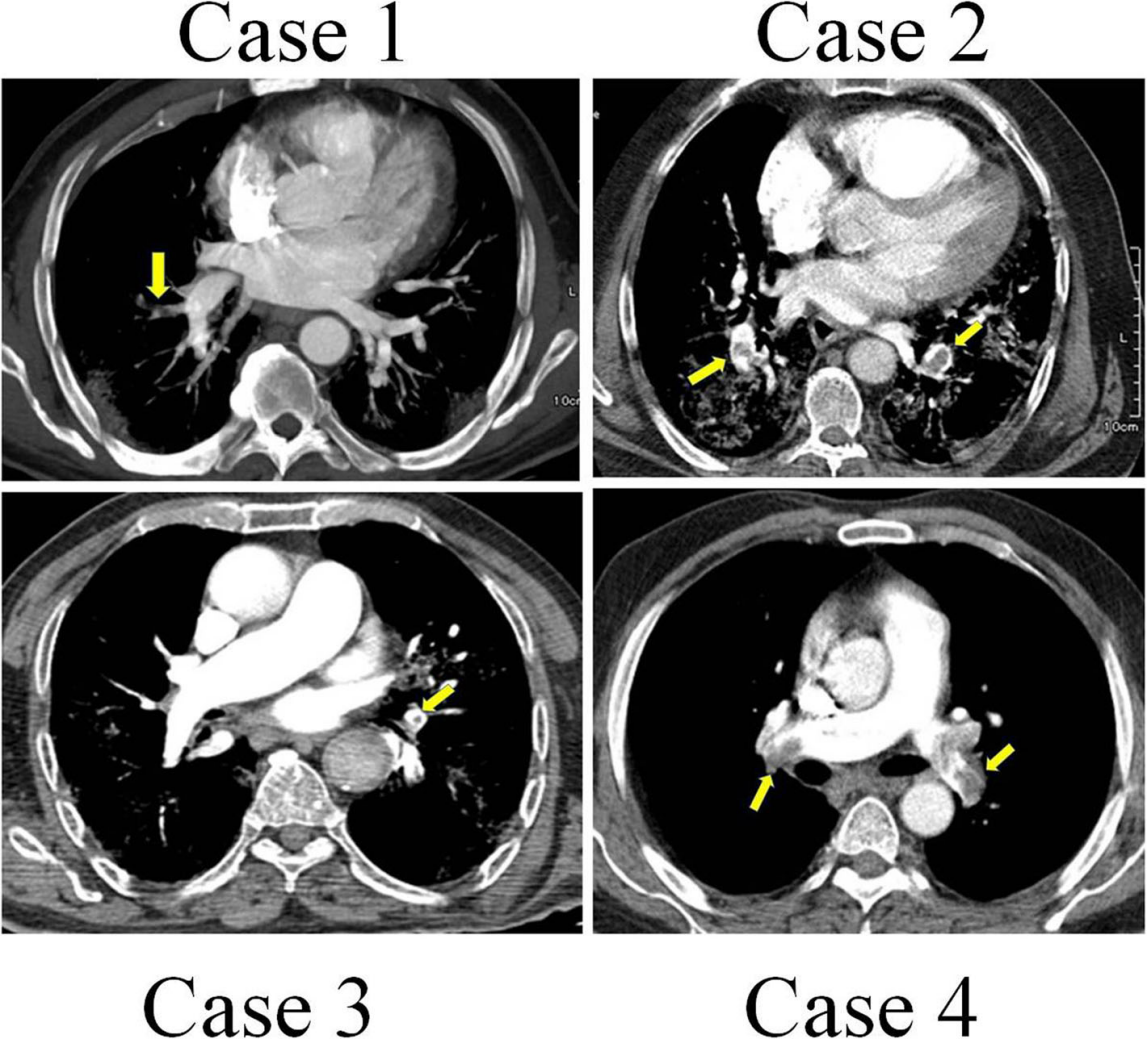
CT angiography images of pulmonary embolism, showing pulmonary artery embolism
